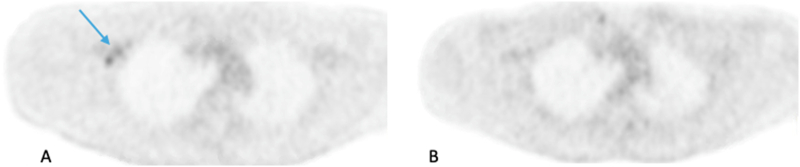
(A) PET-CT showed unenlarged, hypermetabolic lymph node in the right axilla (arrow), thirty-five days after first COVID-19 vaccination. (B) PET-CT showed normalization of previous lymph node activity, seventy-one days after second COVID-19 vaccination.
